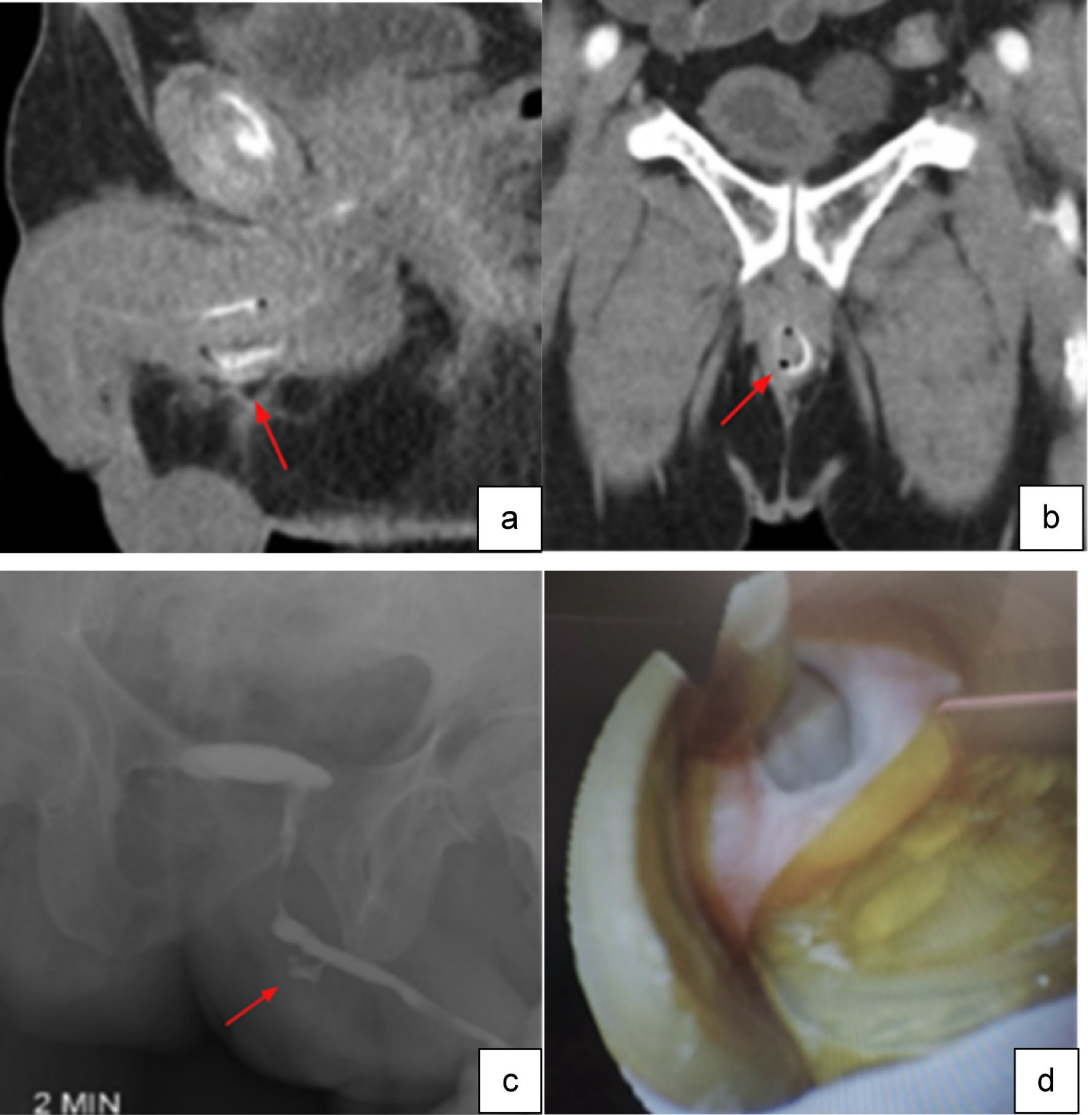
Sagittal (a) and coronal (b) CT images with IV contrast demonstrate foci of gas and fat stranding at the base of the penis concerning for cuff erosion (red arrows). Portable radiograph after retrograde urethrogram (c) shows contrast extravasation at anterior penile urethra in the region of foci of gas on CT (red arrow). Intraoperative cystoscopic view (d) of circumferential artificial urinary sphincter erosion
